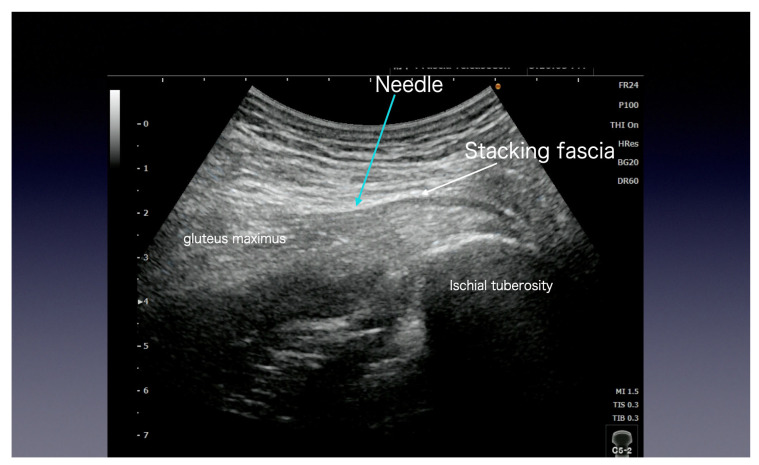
Representative ultrasound image of a local twitch event during fascial hydrorelease (FHR). The needle tip (cyan arrow) contacts the stacking fascia (white arrow) between the gluteus maximus and semimembranosus, with the local twitch centered on the stacking fascia. Acquired with a Konica Minolta linear probe (C5-2/L18-4, FR 24, P 100, THI On, HRes, BG 20, MI 1.5, TIS 0.3, TIB 0.3).
